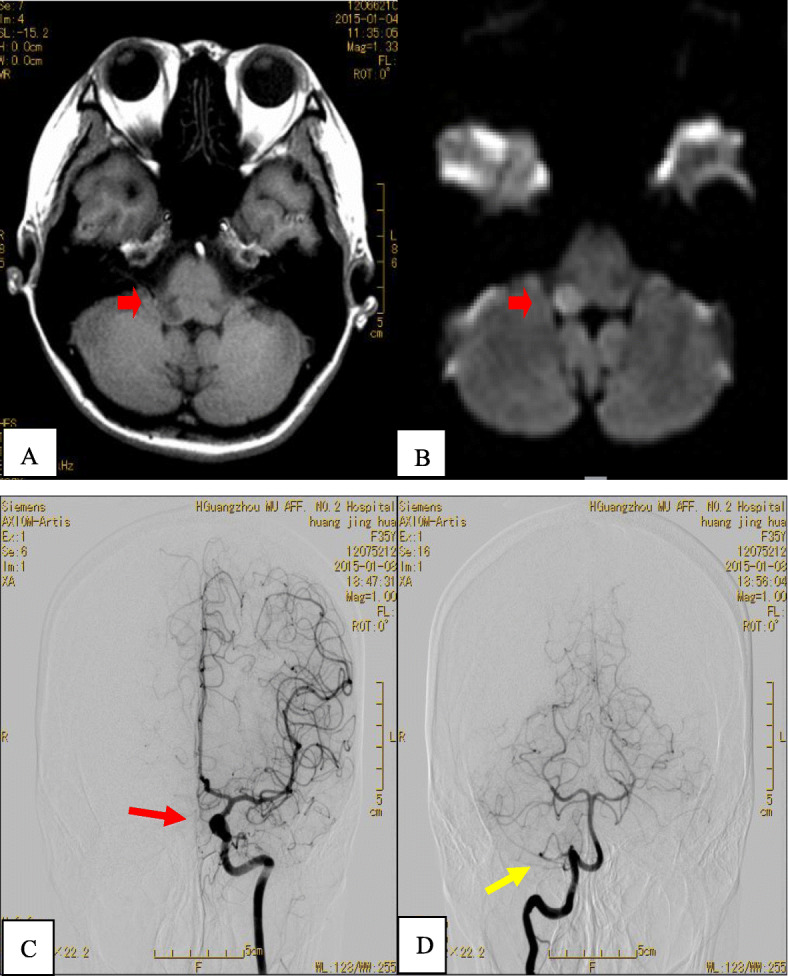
T1-weighted Magnetic resonance imaging (a) and diffusion-weighted imaging (b) showed subacute infarction (red arrow) in the right lateral medullar. Digital subtraction cerebral angiography showed an aneurysm in C2 segment of left internal carotid artery, about 6 mm × 5 mm, with smooth edge (red arrow) (c). Right posterior inferior cerebella artery could be seen clearly on DSA (yellow arrow) (d)
